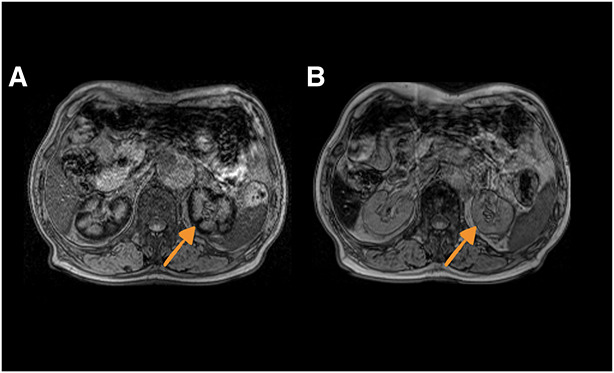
Magnetic resonance imaging shows changes in renal cortical signal intensity on T2*-weighted sequences. The images on the left (A) and right (B) were obtained before and after the repeat surgery, respectively. The left image shows an accentuated decrease in the cortical signal intensity on T2*-weighted sequences. The right image shows a diminishing signal change.
